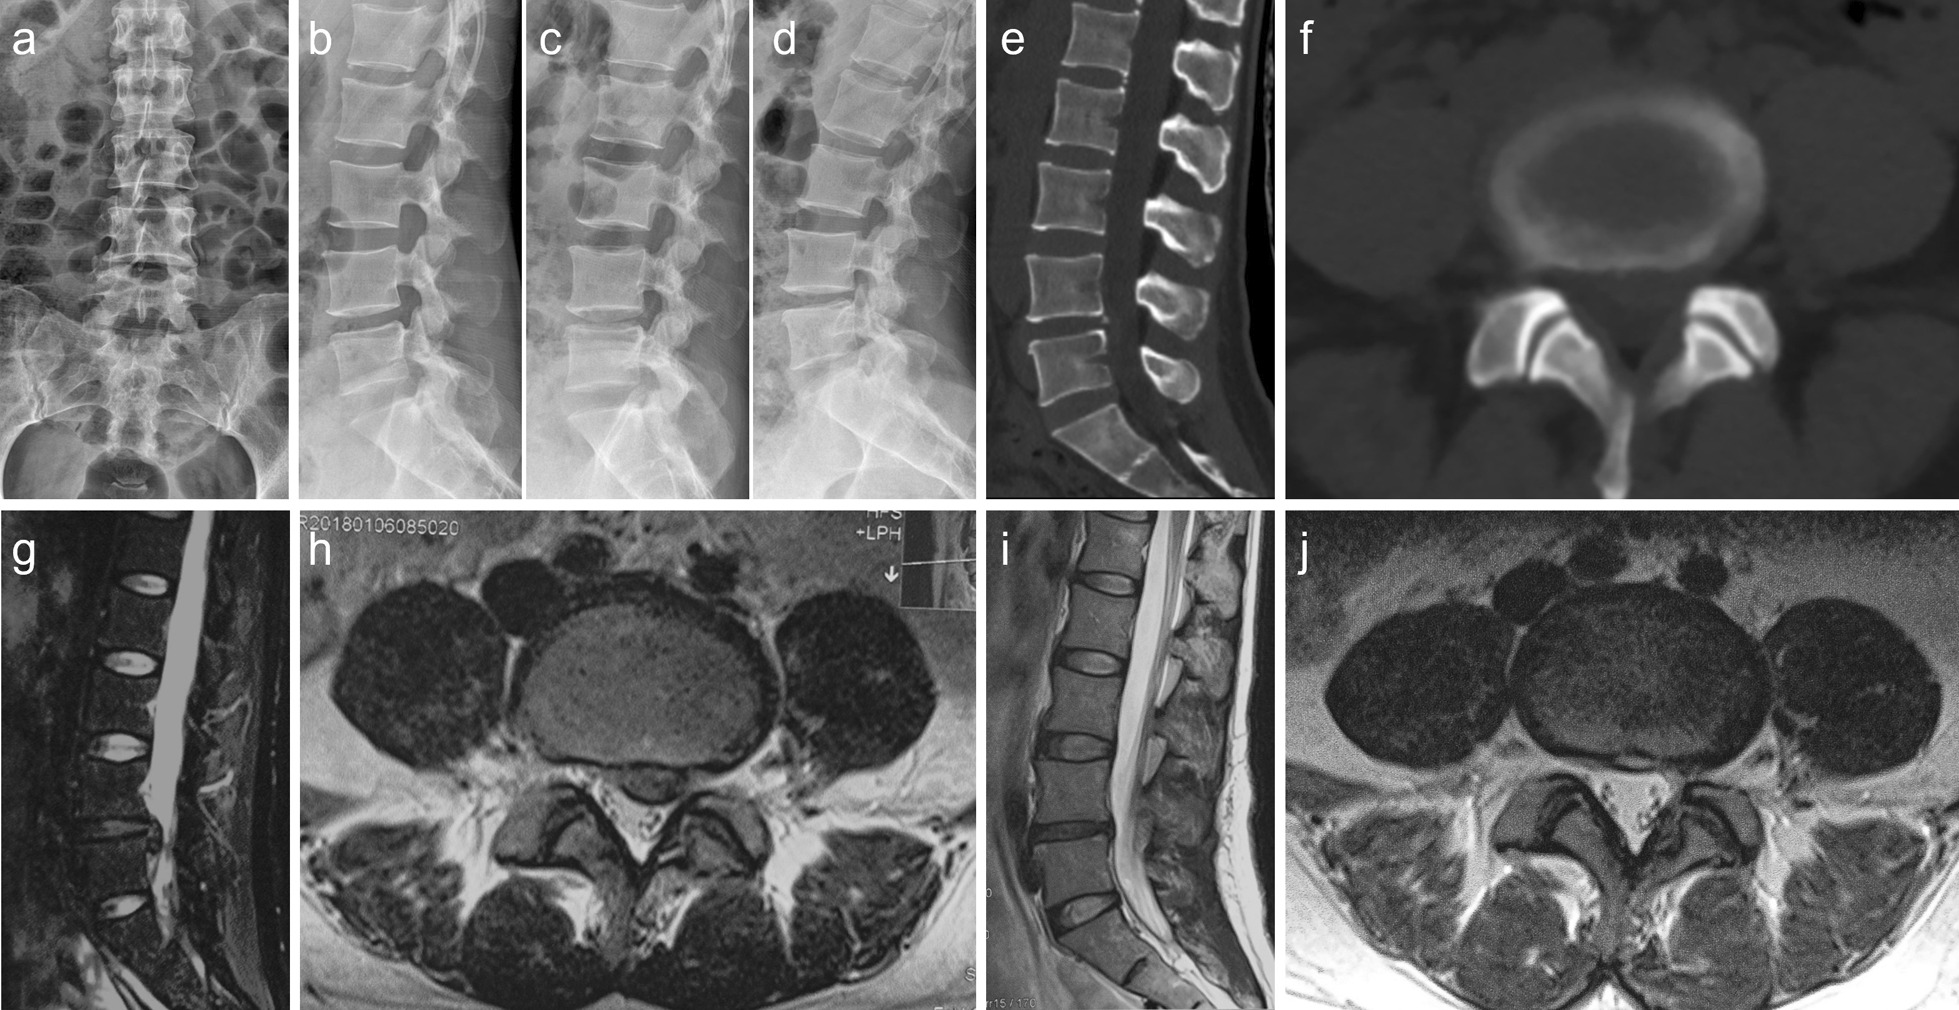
A 36-year-old female with highly downward-migrated disc herniation was treated with percutaneous endoscopic interlaminar discectomy. The patient mainly complained of radiating pain in the left lower limb. The degree of prominence is high grade. a, b Anterior and lateral lumbar radiographs; c, d Dynamic lumbar radiographs; e, f Computed tomography scan of the lower lumbar spine; g, h Magnetic resonance imaging (MRI) of the lumbar spine: Herniated disc at the L_4-5_ segment, nucleus pulposus migration to the posterior edge of L_5_; i, j MRI revealing that the L_4-5_ herniated disc and free nucleus pulposus had been removed
